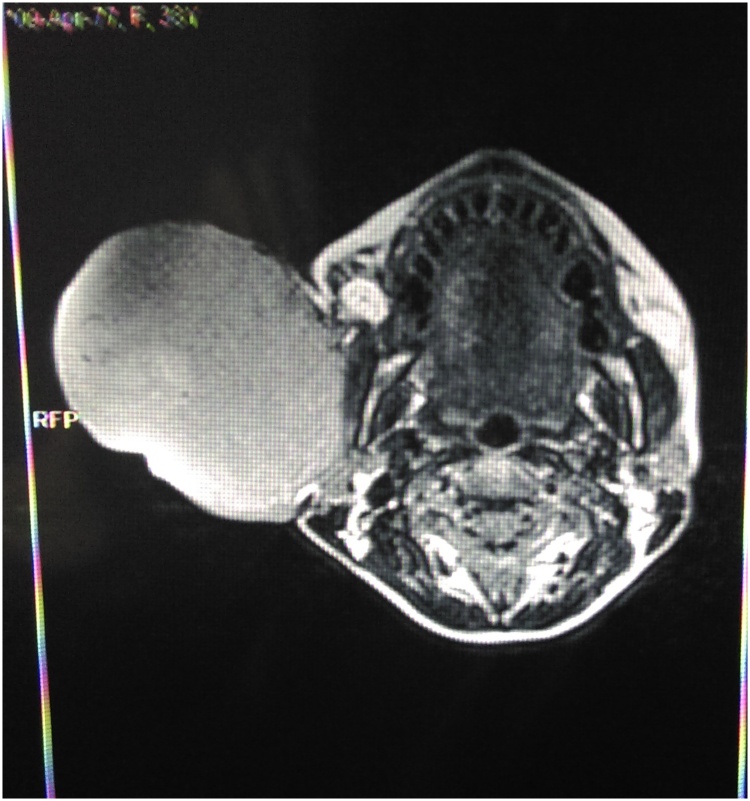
MRI scans showing a right parotid mass.
